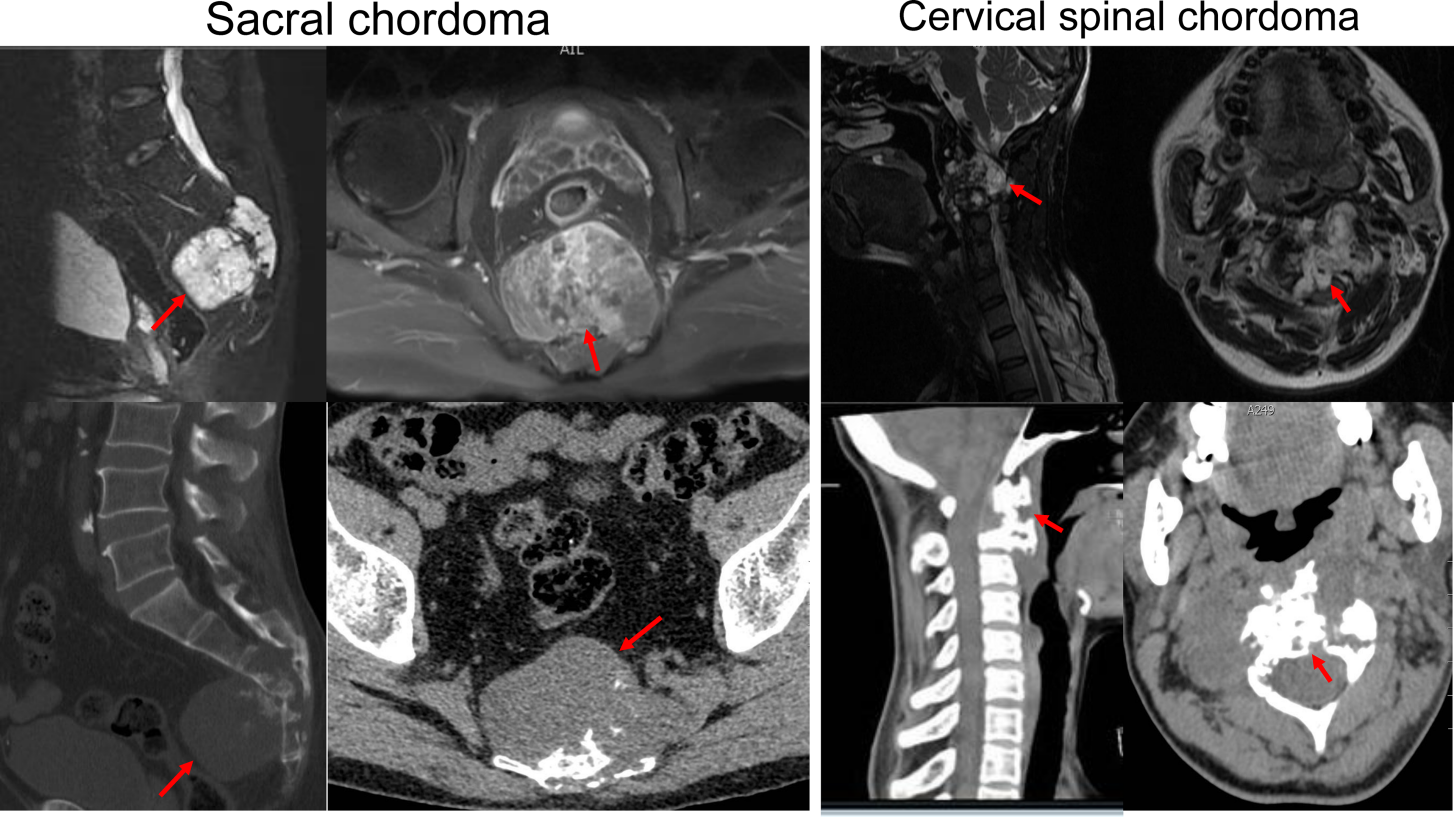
Representative images of MRI and CT scan of sacral and cervical chordoma. The red arrows indicate the location of chordoma.
